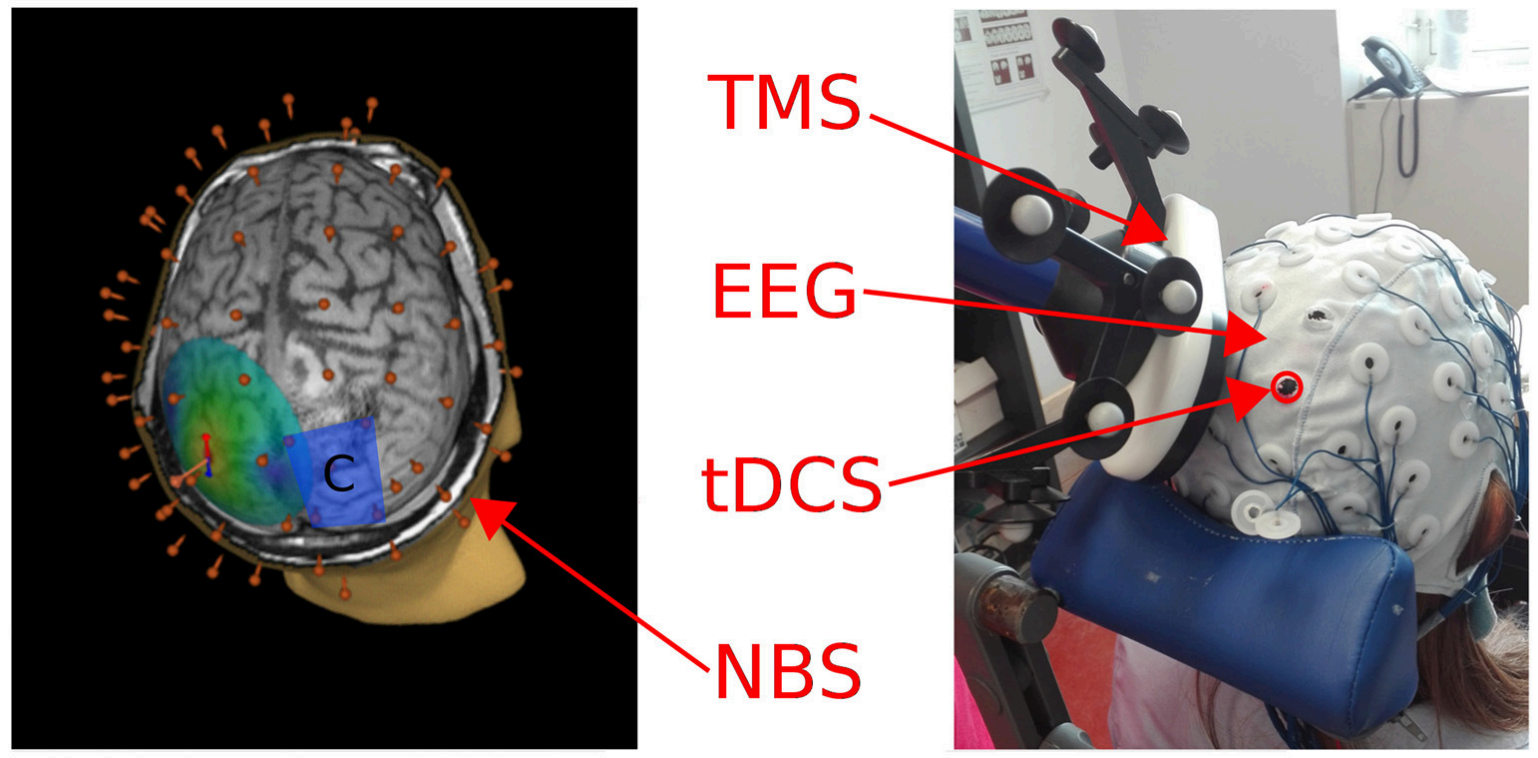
Left: A 3D reconstruction of the individual MRI is depicted, showing the electrical field induced by TMS on the left parietal cortex. The blue rectangle represents the cathode patch position over the right parietal cortex, the 60 red points correspond to the position of the EEG cap electrodes. Right: A picture of the experimental setting used to deliver tDCS and TMS concurrently while recording EEG (during-tDCS condition).
